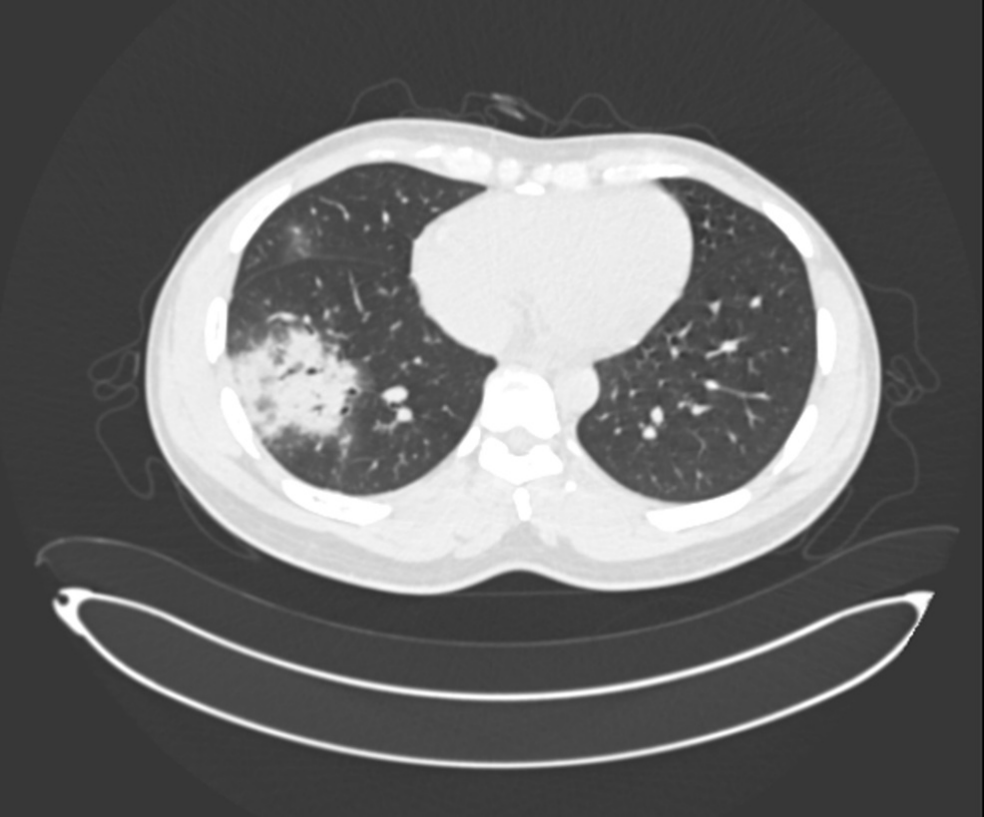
HRCT features of an atypical pneumonic with less than 10% parenchymal involvement.
